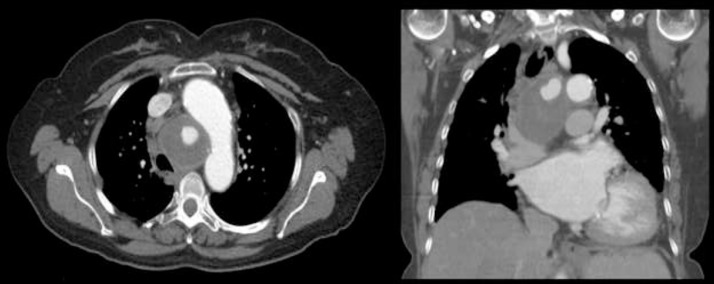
CT angiogram confirmed peripherally thrombosed bronchial artery pseudoaneurysm.
